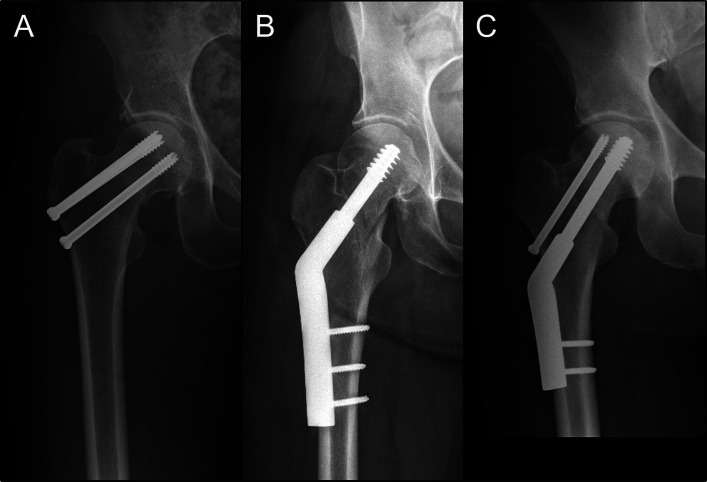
Anteroposterior radiographs showing the internal fixation implant options utilized in the present study, including cannulated screws (Fig. 1-A), a dynamic hip screw (Fig. 1-B), and a dynamic hip screw plus an antirotation screw (Fig. 1-C).
